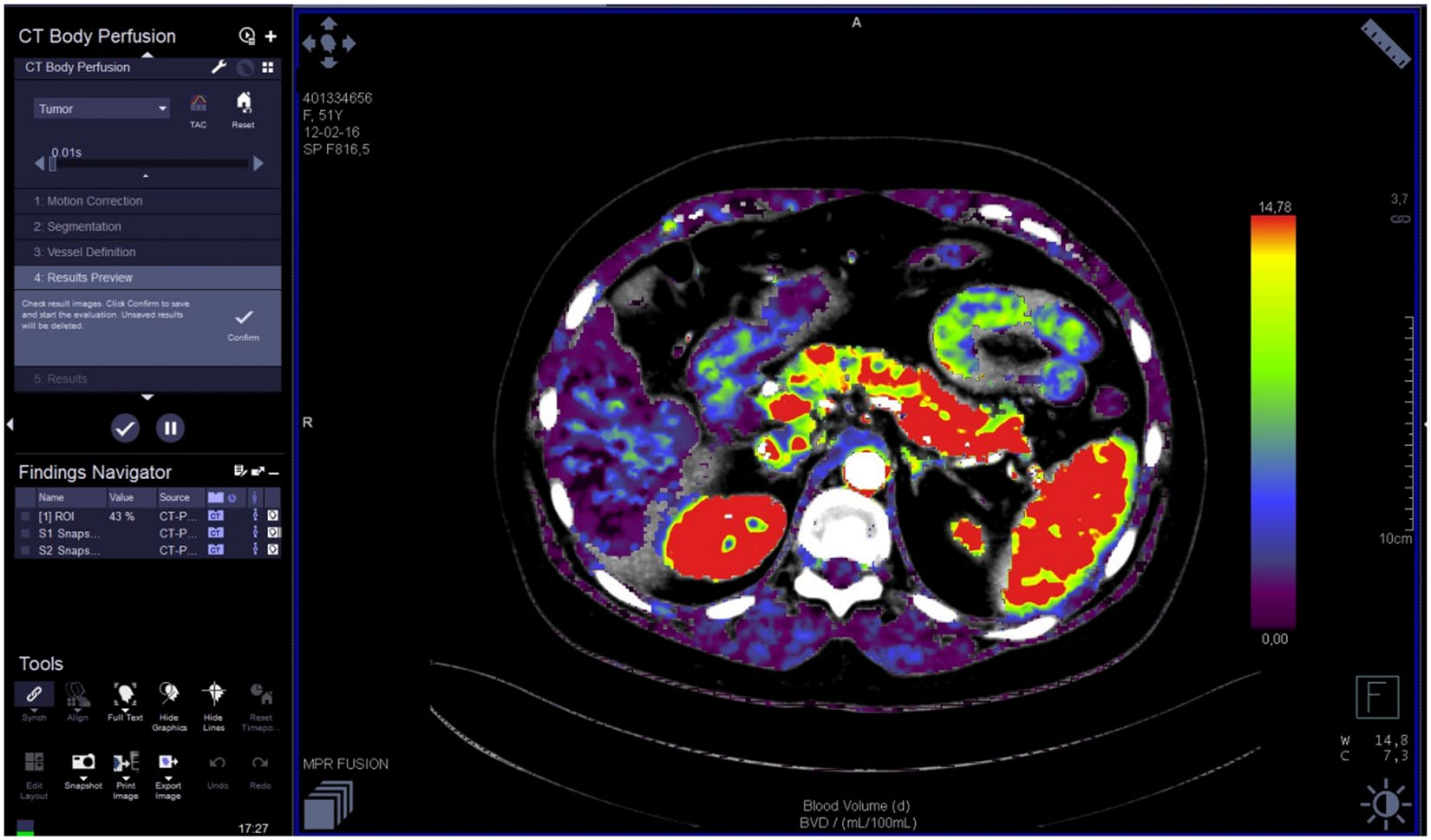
Perfusion CT: image processing.
